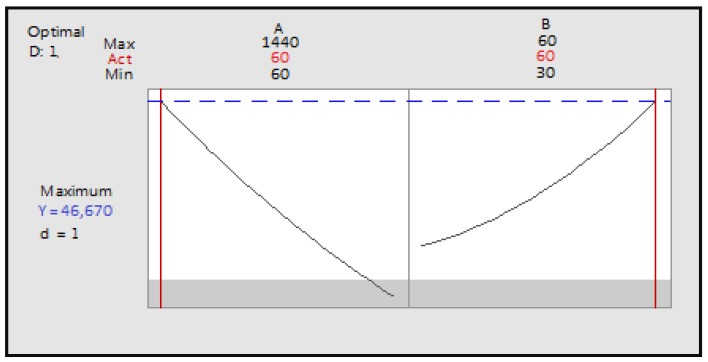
Optimization of the two factors maceration time and ultrasonication time as a function of desirability; A: maceration time; B: ultrasonication time.
